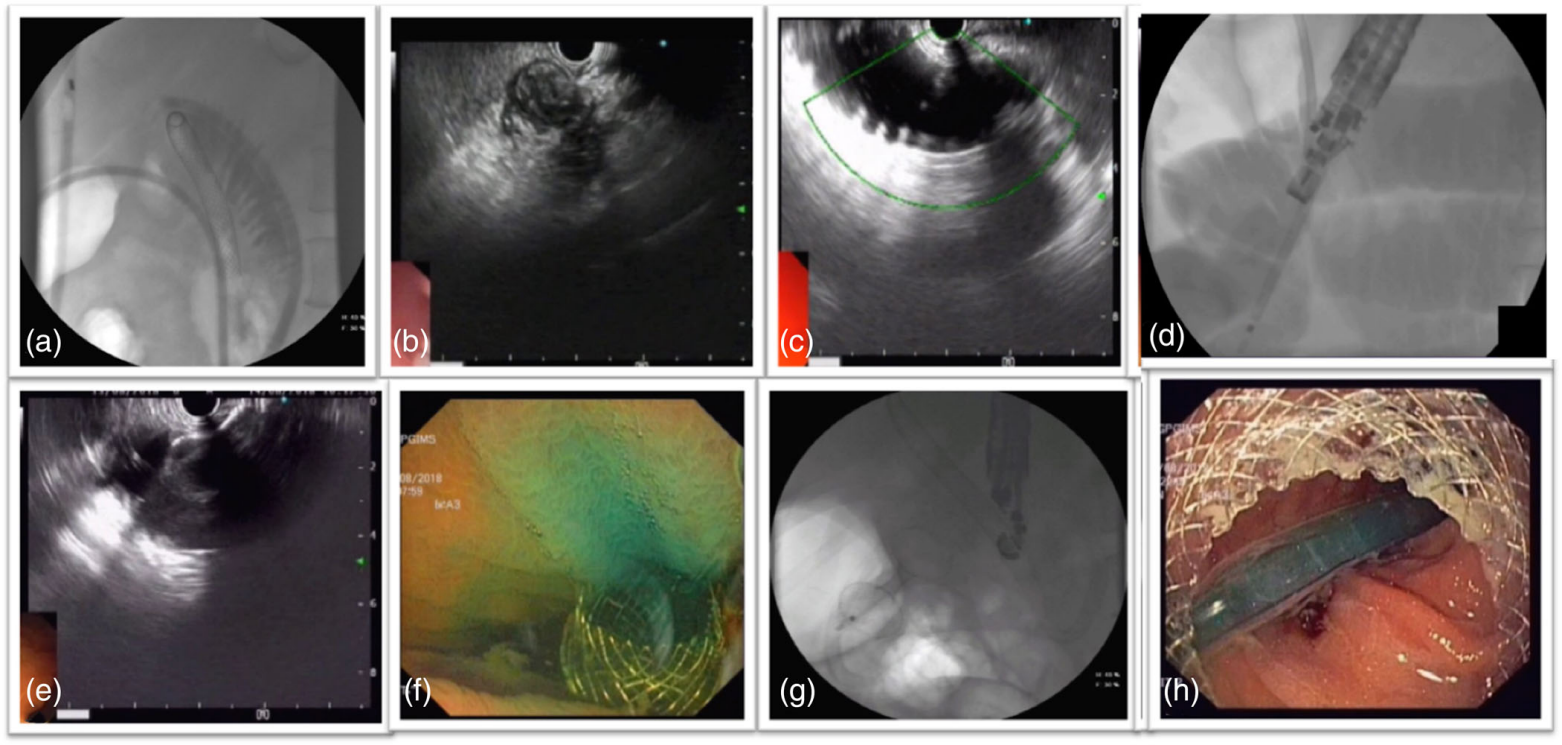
(a) Placement of a nasojejunal tube. (b) Collapsed small bowel loop seen on endoscopic ultrasound. (c) Distension of small bowel loop with normal saline with methylene blue and contrast. (d) Direct puncture of the small bowel loop with Hot Axios stent. (e) Release of the distal flange of Hot Axios stent. (f) Release of the proximal flange of the stent with blue‐colored fluid seen in the stomach. (g) Stent with a waist seen immediately after deployment. (h) Small bowel loop seen with the endoscope
